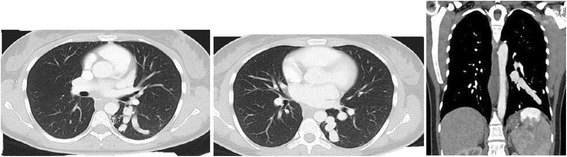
A chest computed tomography scan showing varicose veins of the lower lobe.
